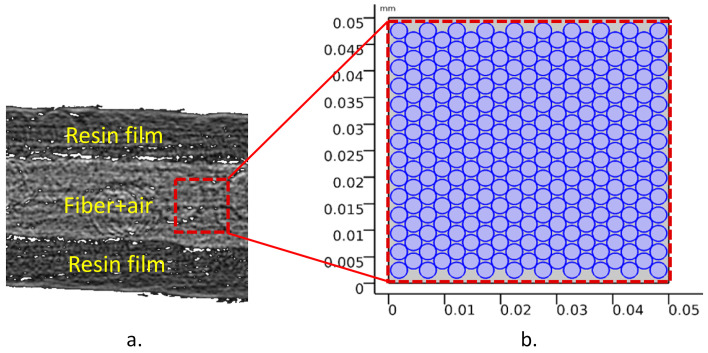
Model domain for numerical permability characterization: (a) CT image, (b) representative numerical model domain with the dry fiber bed with fibers (dark circles) and air (light areas).
